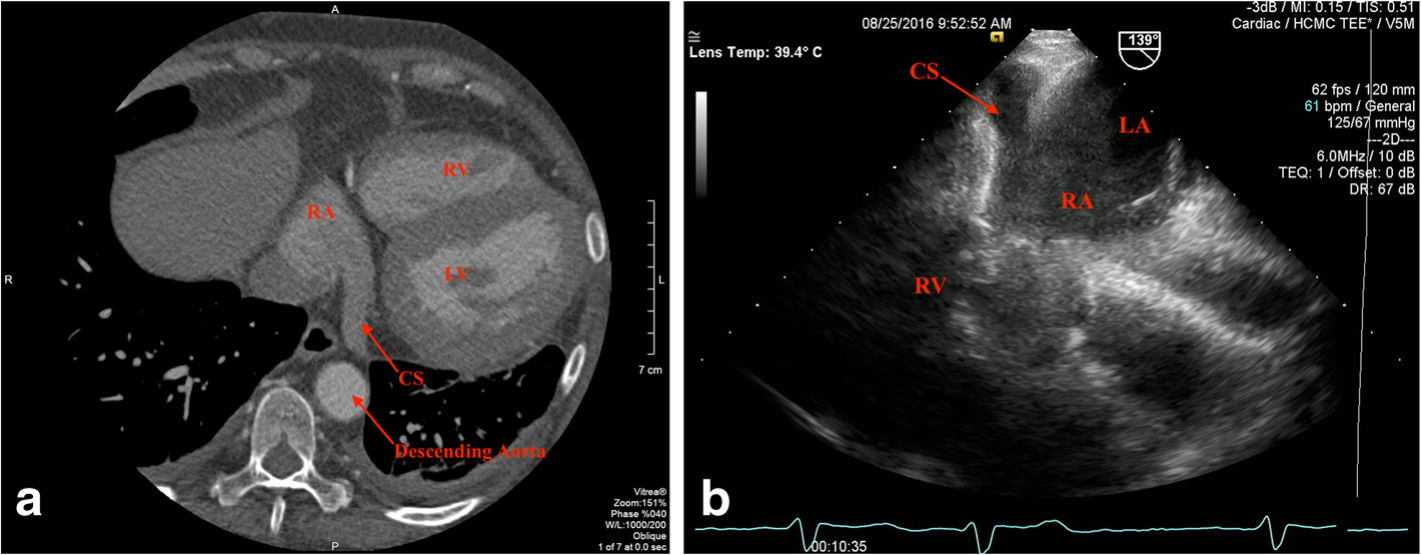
a Gated cardiac computed tomography at the coronary sinus level showing patent coronary sinus with resolution of the prior filling defect after 3 months of systemic anticoagulation. b Transesophageal echocardiogram at the gastroesophageal junction positioned at 139° confirmed patent coronary sinus. *CS* coronary sinus, *LA* left atrium, *LV* left ventricle, *RA* right atrium, *RV* right ventricle
